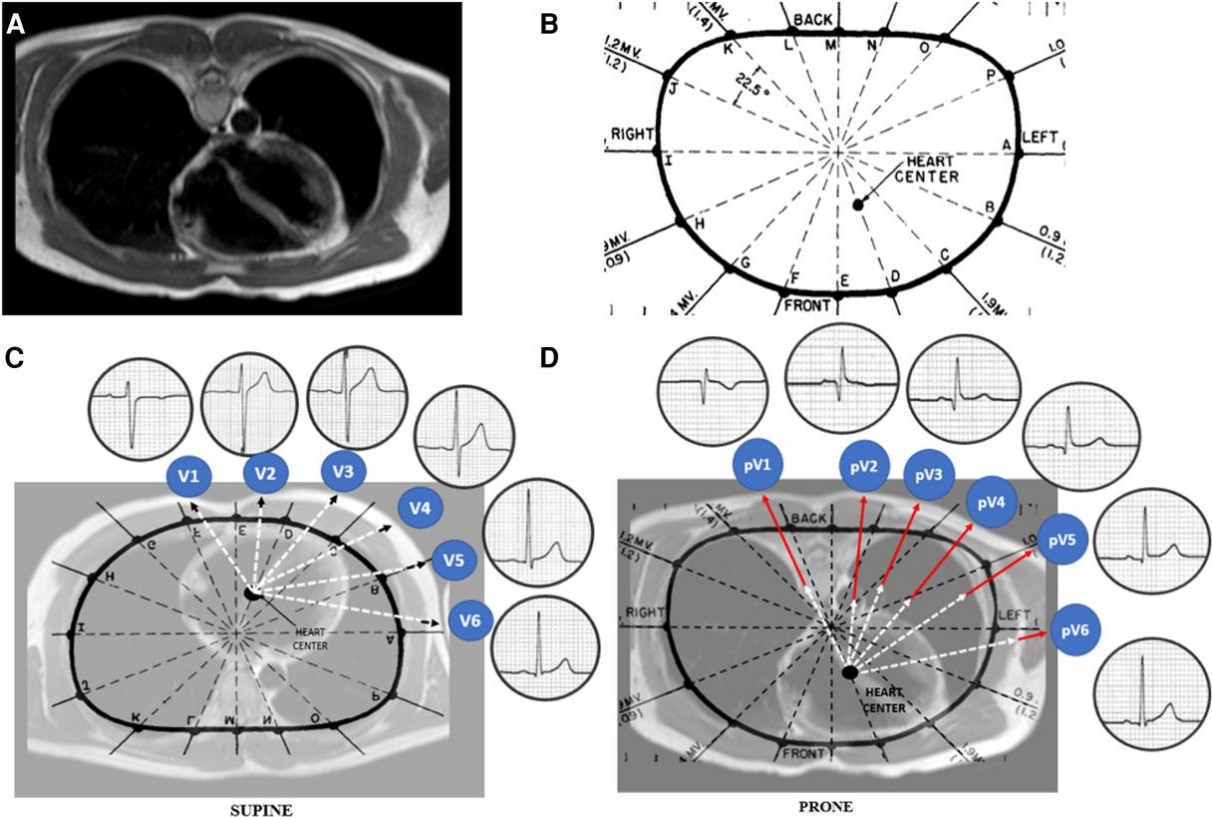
Assessment of the electroanatomic position of the heart. Difference in the distance from the centre of ventricular depolarization of the heart to the precordial electrodes in the supine and in prone position by chest magnetic resonance images. (*A*) Prone chest magnetic resonance imaging of a healthy volunteer. (*B*) Localization of the centre of ventricular depolarization (centre of the heart). (*C*) Measurement of the distance from the centre of the heart to the standard supine lead position (dotted arrows); see QRS morphology in supine position. (*D*) Measurement of the distance from centre of the heart to posterior prone lead position (dotted arrows represent the distance measured to standard lead position when supine; straight arrows show the difference in distance between anterior and posterior lead placement); see QRS morphology in prone back position. [Reproduced from ‘Frank E. Determination of the electrical centre of ventricular depolarization in the human heart. *Am Heart J* 1955;49:670–92’ with permission of the publisher].
